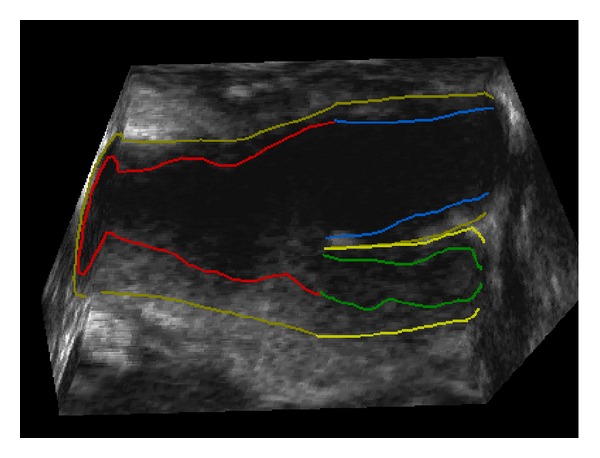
Three-dimensional ultrasound carotid artery longitudinal view in clinical trials [40]. Both baseline and follow-up 3D images, constructed from the set of 2D frames, were examined simultaneously to visually match the bifurcation (BF) points in both images by an operator blinded to time point and treatment. Each 3D US image was manually segmented starting from the bifurcation point extending into around 10–15 mm of common carotid artery (CCA) and about 10 mm into internal carotid artery (ICA) at 1 mm interval perpendicular to the artery axis; refer to Figure 3. This study was only carried out on the CCA, since the focus was on stroke risk.
